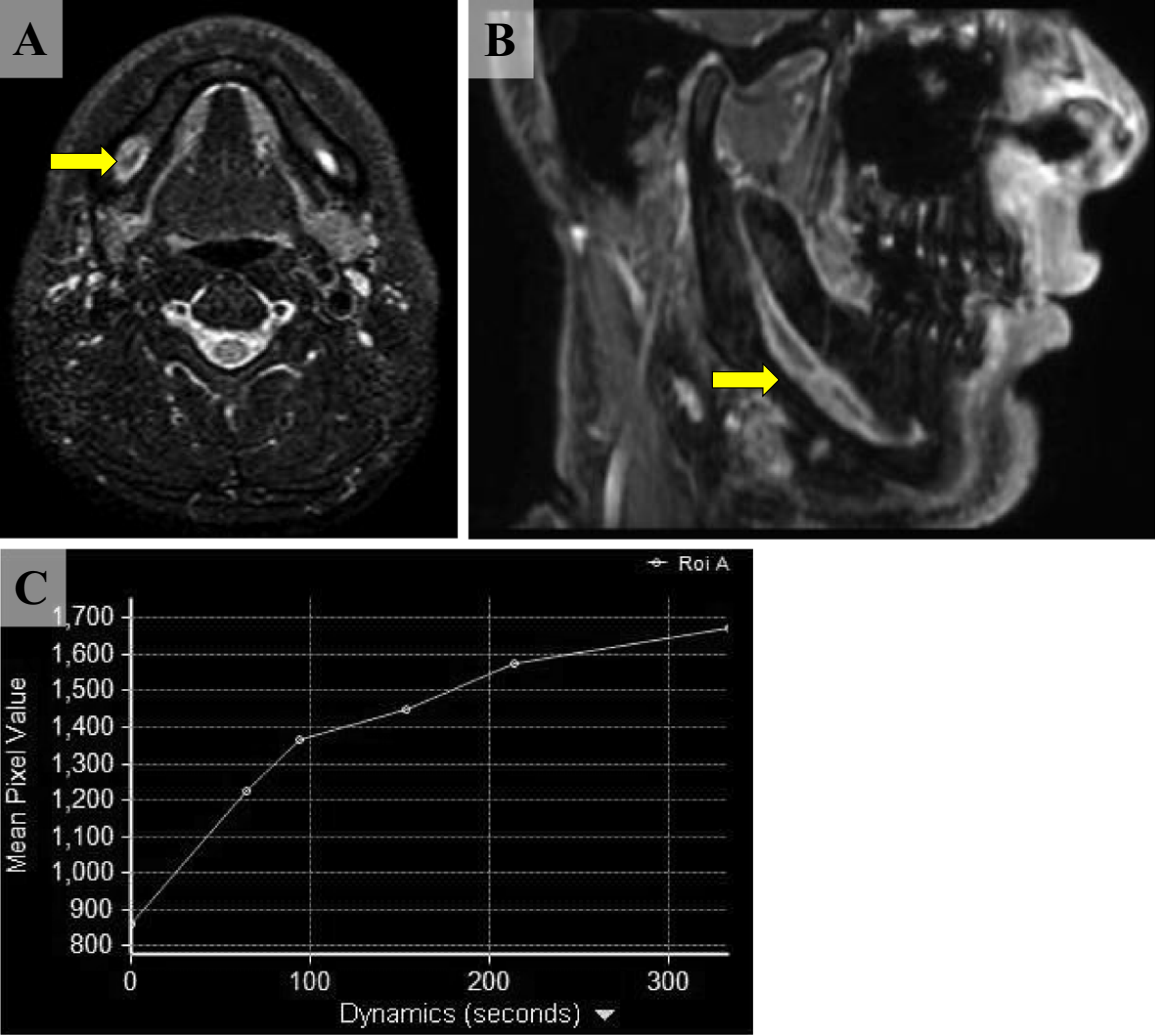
MRI findings. a Horizontal section. An irregular high signal in the STIR image was recognized (yellow arrow). b Sagittal sections. Dilation of the right mandibular canal was observed around the mandibular foramen (yellow arrow). c Dynamic images showed crescendo enhancement
